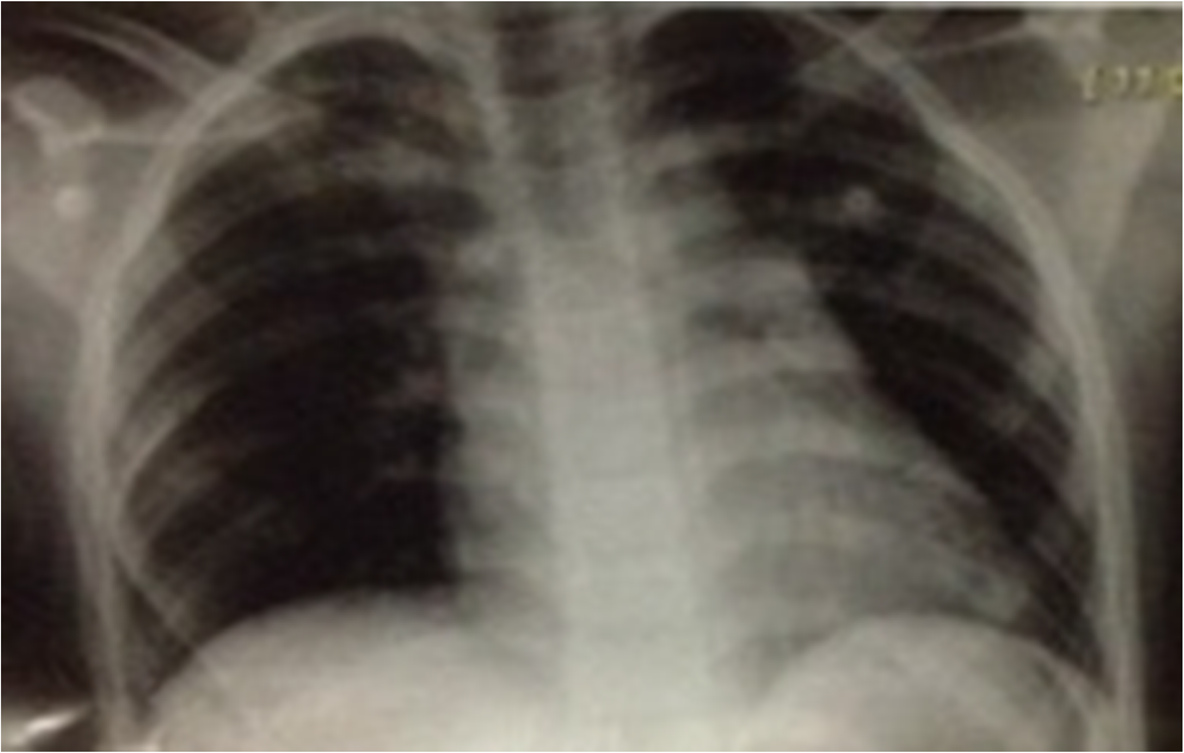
Standard X-ray of the chest (normal)
